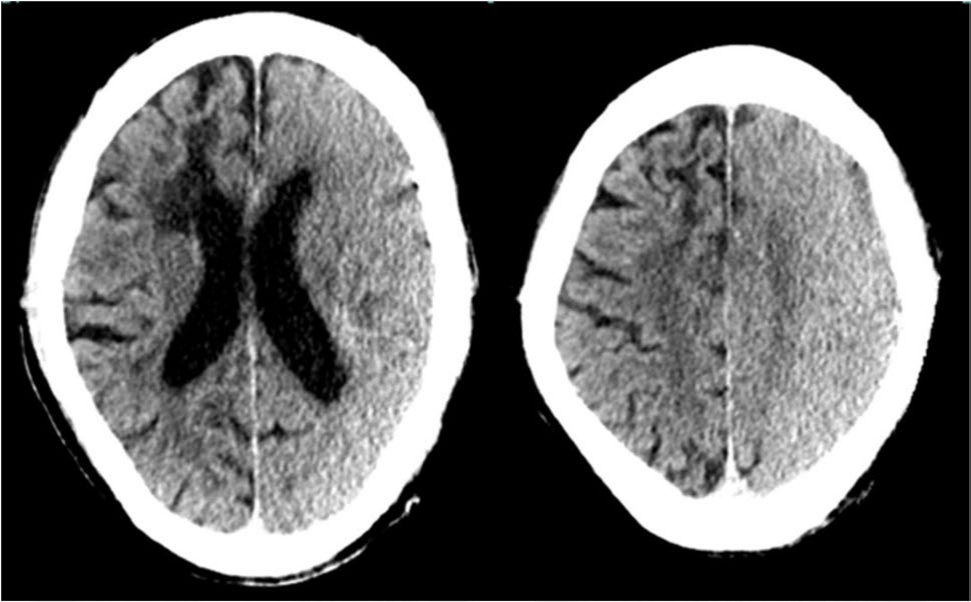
Non-contrast brain CT on POD1. CT showing sulcal effacement and high attenuation in the left hemisphere, indicating cerebral edema and suspected contrast leakage. CT: computed tomography. POD: postoperative day
